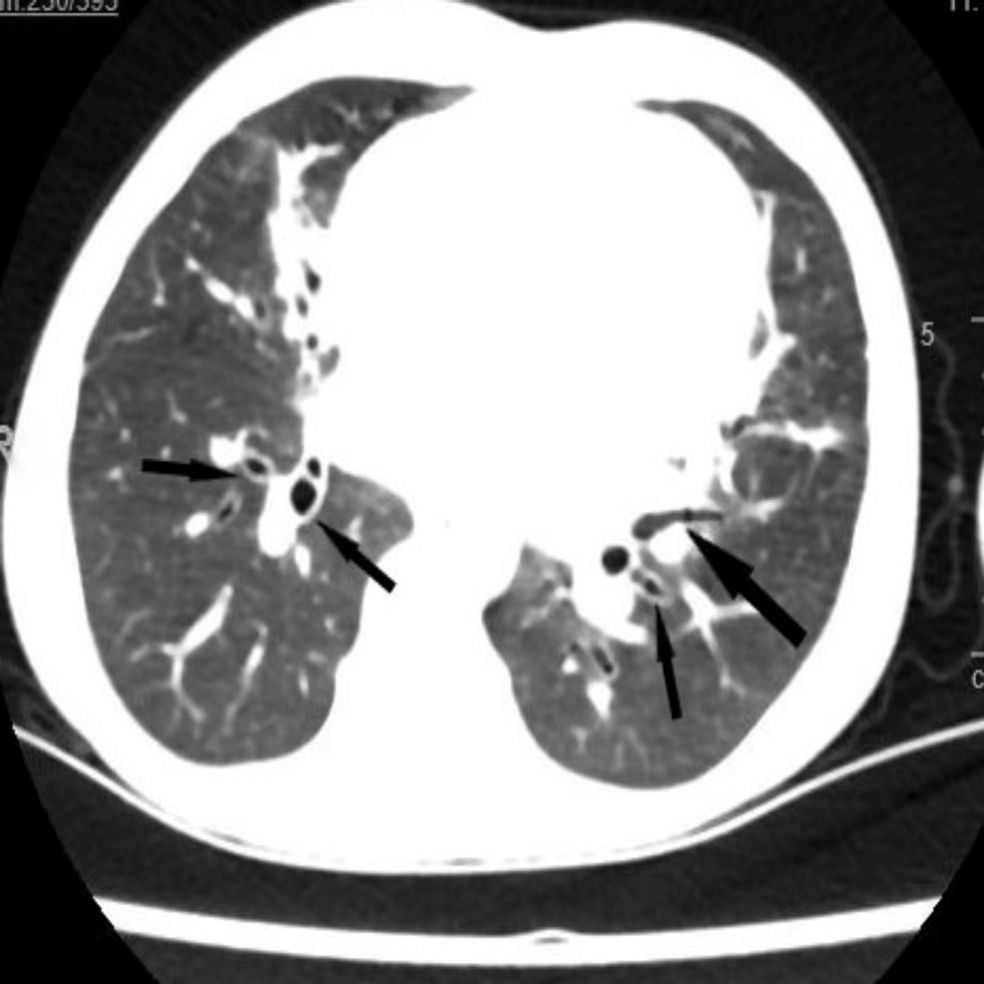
Chest CT scan showed significant tubular bronchiectasis in the upper and lower lobes bilaterally and the left lingular segments (black arrow)
